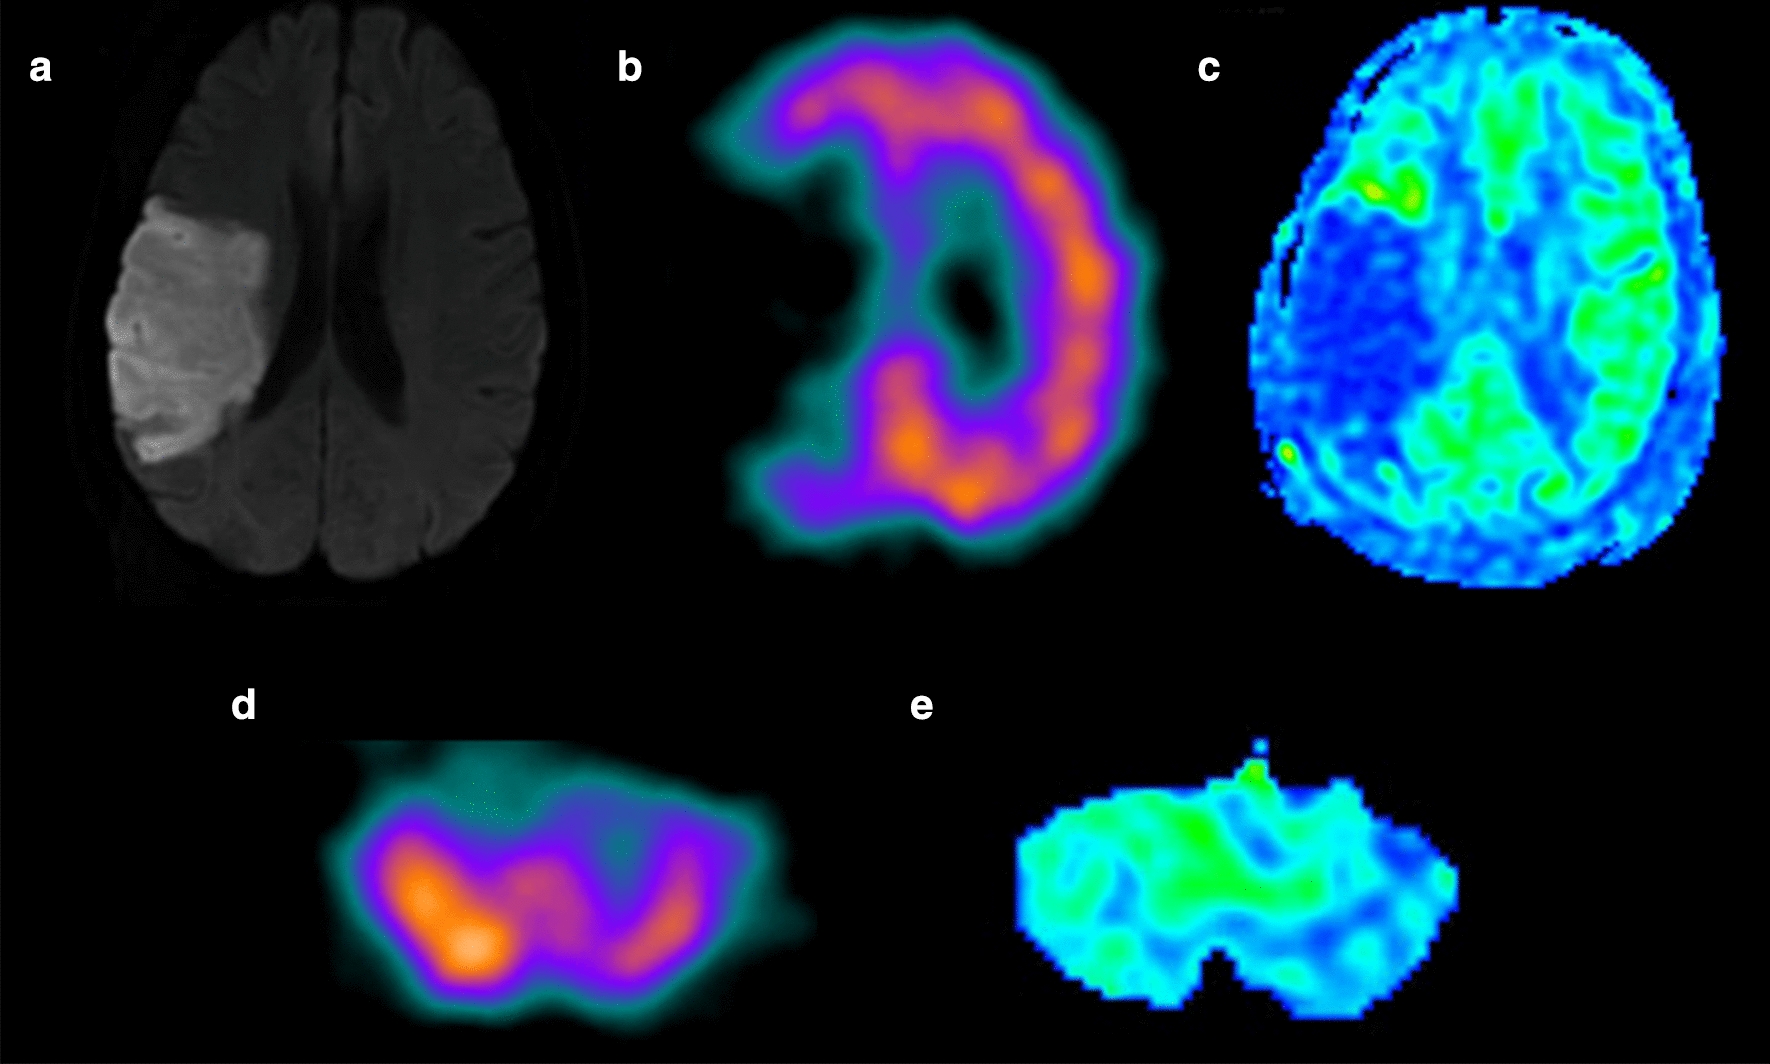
A 62‑year‑old man with a history of sudden onset left-sided weakness for 7 days. a Hyperintensity stroke lesion can be recognized in the right temporal lobe on diffusion weighted image. b SPECT and c ASL maps showing hypoperfusion in the same lesion. d SPECT and e ASL images showing hypoperfusion in the contralateral cerebellum hemisphere
